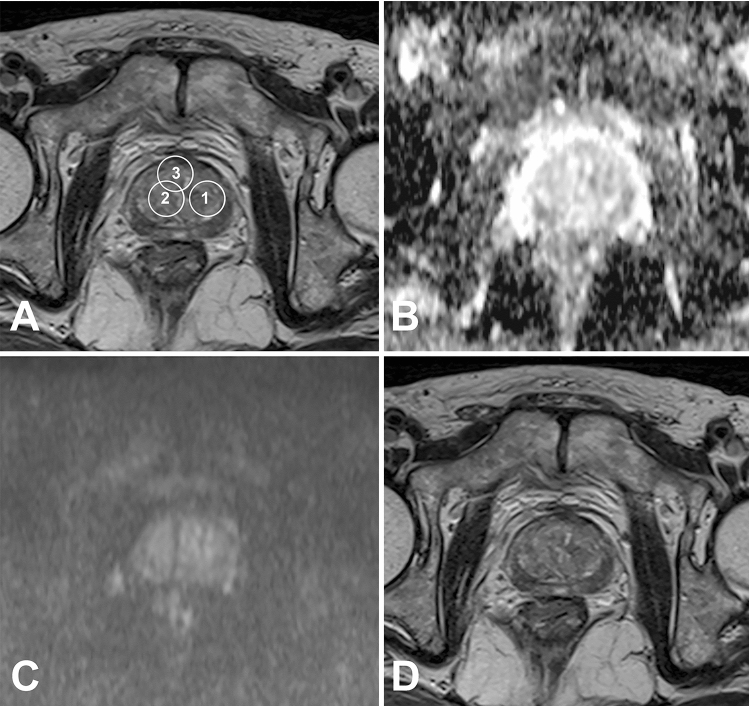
A 74-year-old man; only one core in the left TZa zone (No. 1 in A) had a GS of 4 + 3 = 7. The lesion accounted for 2% of the tissue strips, among which 4 score components accounted for 60% (1.8% of the tissue strips) and 3 score components accounted for 40% (0.8% of the tissue strips). The GS of the two cores from the right TZa and AFS (No. 3 and 2 in A, respectively) is 3 + 3 = 6, accounting for 4% and 1% of the tissue strips, respectively. No lesion with a PI-RADS score ≥ 3 could be found in the MR images, and the ML models misjudged
